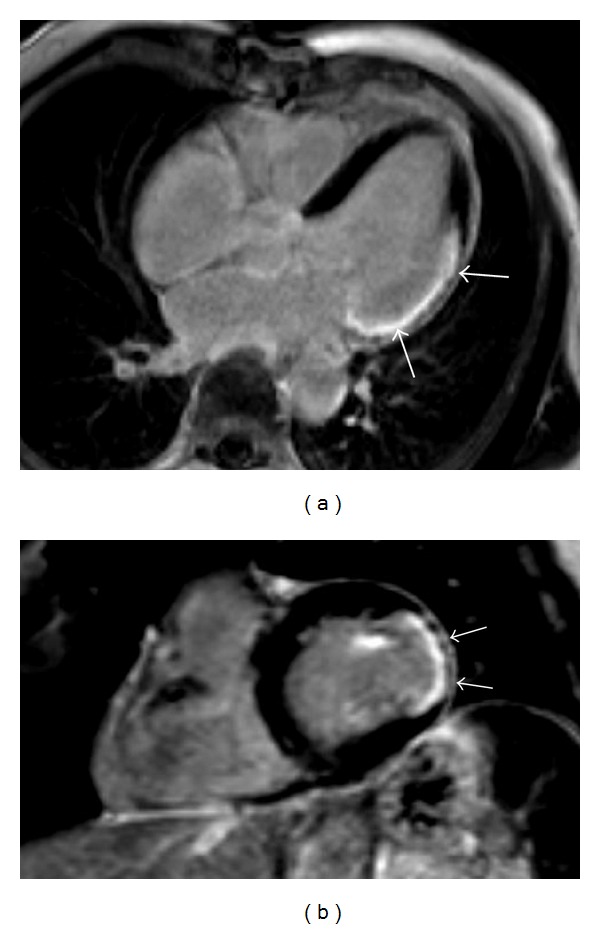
Delayed-enhancement MR images of a patient with chronic lateral myocardial infarction. Both the 3-chamber view (a) and the short-axis view (b) demonstrate the presence of a transmural lateral scar (arrows).
